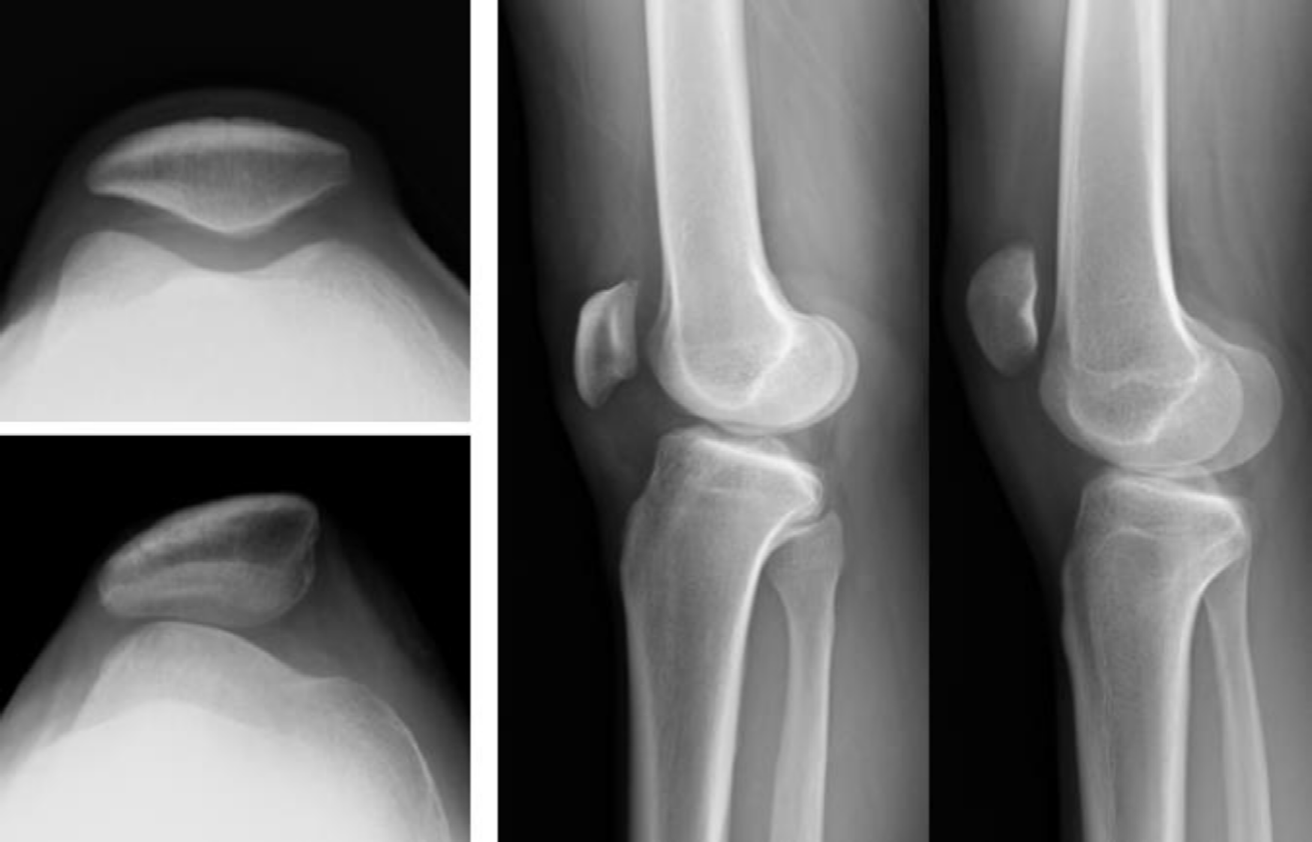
Comparison of normal and abnormal features on radiographs: normal (left upper) or dysplastic trochlear groove (left lower); normal location (middle) or high-riding (patella alta) of the patella (right).
